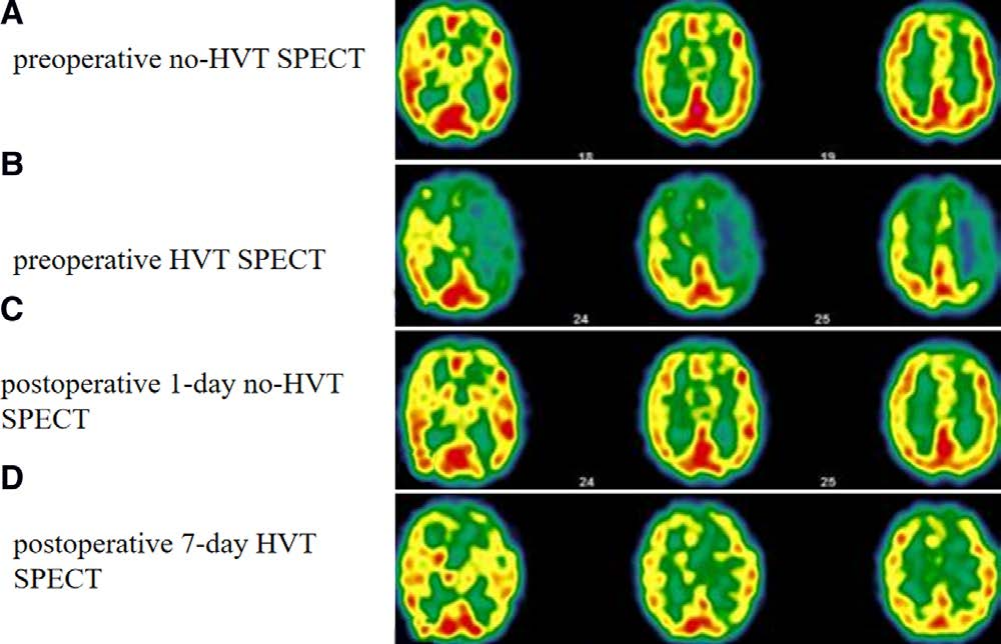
Temporal profile of 123I-IMP-SPECT images showed: (A) decreased CBF in bilateral frontal parietal lobe and right temporal lobe; (B) more decreased CBF in the left frontal, temporal, parietal lobes and basal ganglia than that of the contralateral hemisphere; (C) no obvious changes compared to preoperative no-HVT SPECT; (D) a significant improvement, compared to preoperative no-HVT SPECT. CBF = cerebral blood flow, HIT = hyperventilation test, SPECT = single photon emission computed tomography.
